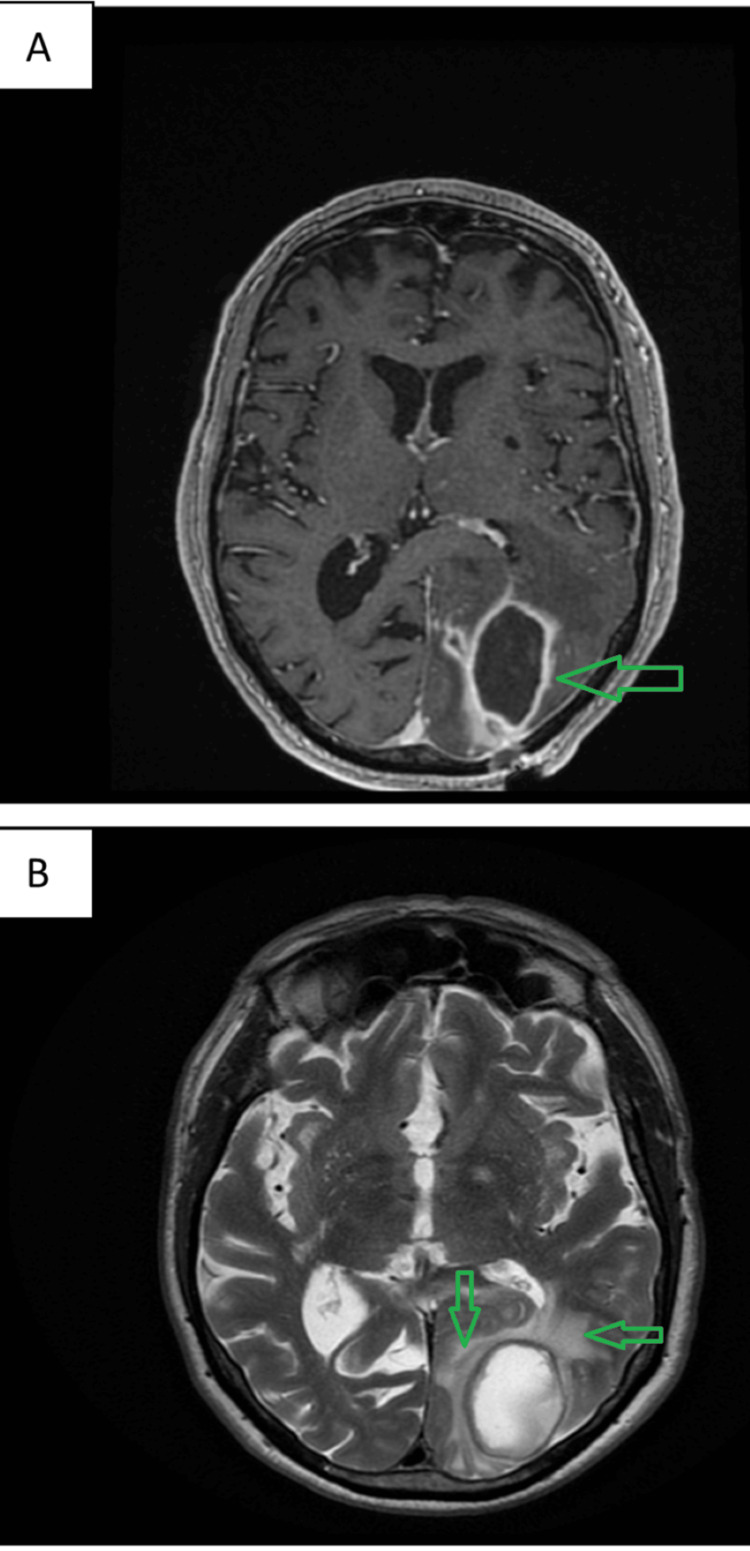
MRI of the brain A) T1 with gadolinium, showing a ring-shaped contrast enhancement suggestive of a brain abscess (arrow); B) T2 showing perilesional oedema (arrows). MRI, Magnetic resonance imaging
